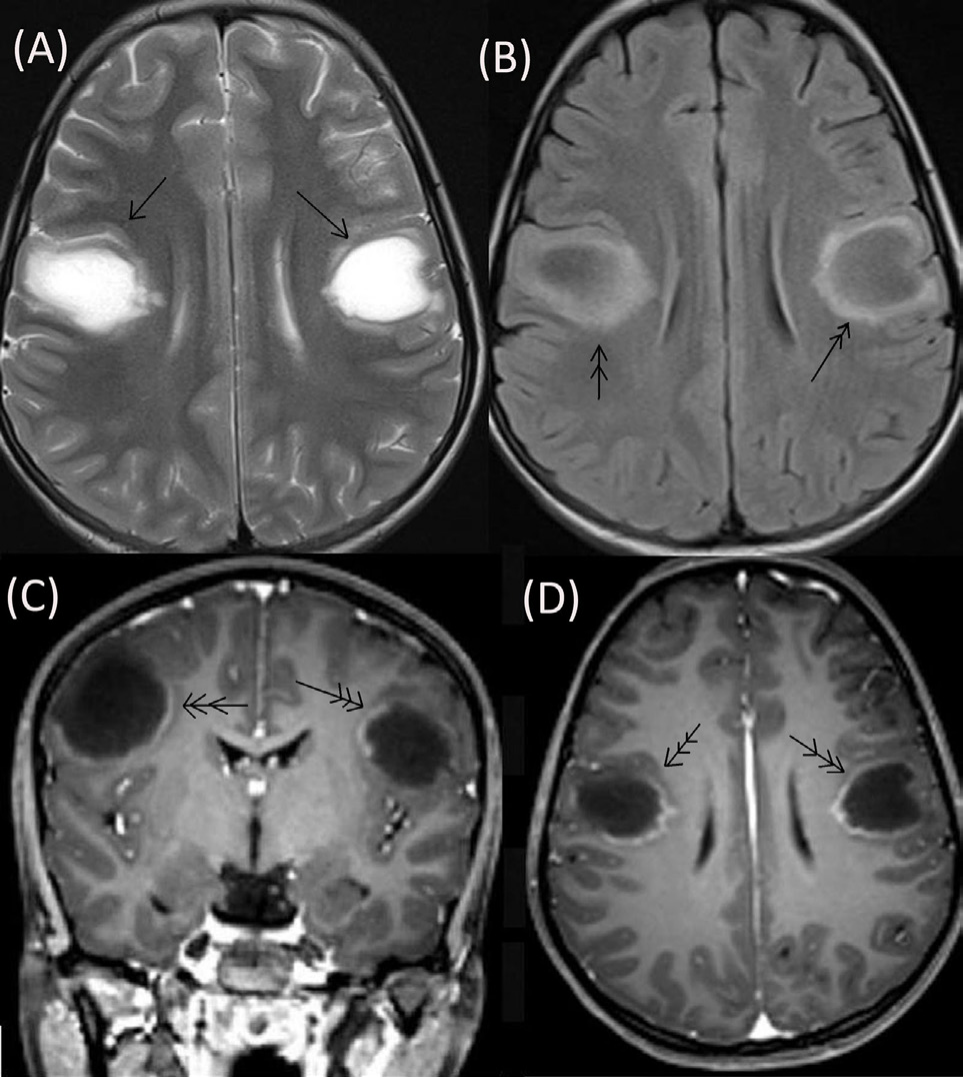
Repeat MRI brain showing bilateral frontal–parietal hyperintensities on T2 sequence (single arrow) with central hypointensity on FLAIR (double arrow) (A,B). On contrast injection incomplete ring enhancement was seen (triple arrow) (C,D).
